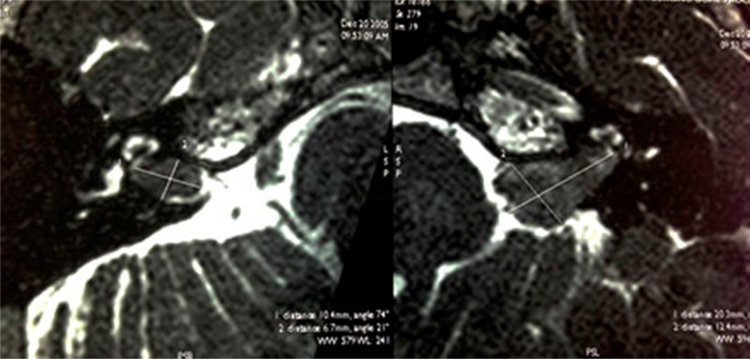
T2-weighted axial MRI before radiotherapy, showing an expansive lesion measuring 1.0 cm × 0.7 cm × 0.7 cm and occupying the right internal auditory canal (T1 according to the classification of Hannover) and another injury of the same kind measuring 2.0 cm × 1.2 cm × 0.7 cm on the left (Hannover T3a).
